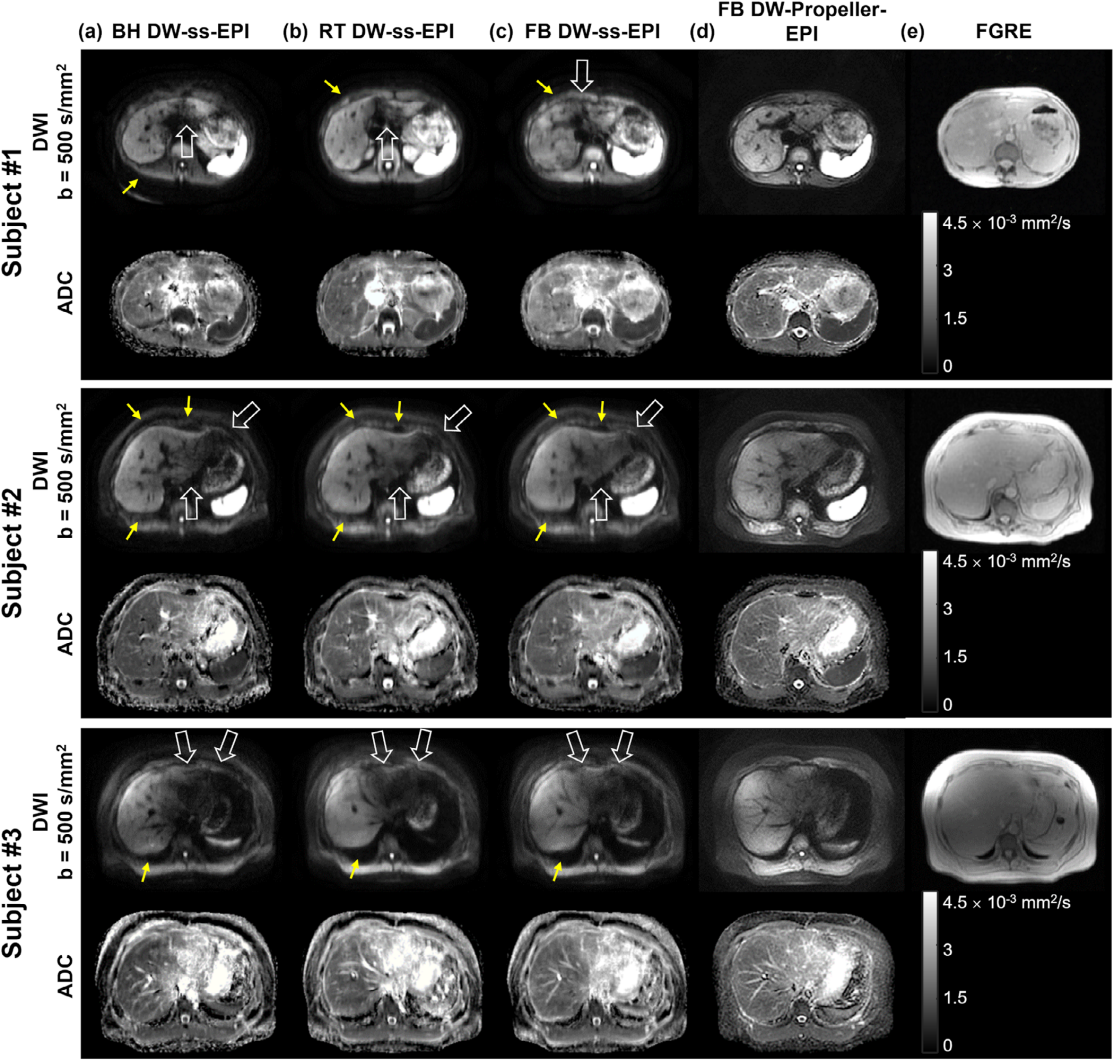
Liver diffusion images (at b‐value = 500 s/mm^2^) and corresponding ADC maps obtained from three subjects (Subject #1: a 51‐year‐old woman, Subject #2: a 63‐year‐old woman, and Subject #3: a 42‐year‐old man) are shown, acquired using four techniques: (a) breath‐holding diffusion‐weighted single‐shot echo‐planar imaging (BH DW‐ss‐EPI), (b) respiratory‐triggering diffusion‐weighted single‐shot echo‐planar imaging (RT DW‐ss‐EPI), (c) free‐breathing diffusion‐weighted single‐shot echo‐planar imaging (FB DW‐ss‐EPI), and (d) free‐breathing diffusion‐weighted Propeller echo‐planar imaging (FB DW‐Propeller‐EPI). (e) Fast gradient‐echo (FGRE) images, serving as the gold standard for geometric fidelity, are also displayed for each case. Yellow arrows highlight severer geometric distortion in DWI images obtained with three routine DW‐ss‐EPI techniques. In contrast, DWI images obtained with FB DW‐Propeller‐EPI technique exhibit geometric fidelity visually comparable to FGRE images. In addition, as indicated by the white arrows, DWI images obtained with three routine DW‐ss‐EPI techniques show more signal loss in the left liver lobe compared with FB DW‐Propeller‐EPI technique. For BH DW‐ss‐EPI, RT DW‐ss‐EPI, FB DW‐ss‐EPI and FB DW‐Propeller‐EPI techniques, average geometric fidelity scores from two raters were: 2, 3.5, 4, and 5 for subject #1; 3, 4, 2.5, and 5 for subject #2; 4, 3, 2, and 5 for subject #3. Average scores regarding signal homogeneity in the left lobe for the four techniques were: 3, 2, 4, and 5 for subject #1; 2, 3, 4, and 5 for subject #2; 3, 2, 3, and 4 for subject #3, respectively.
